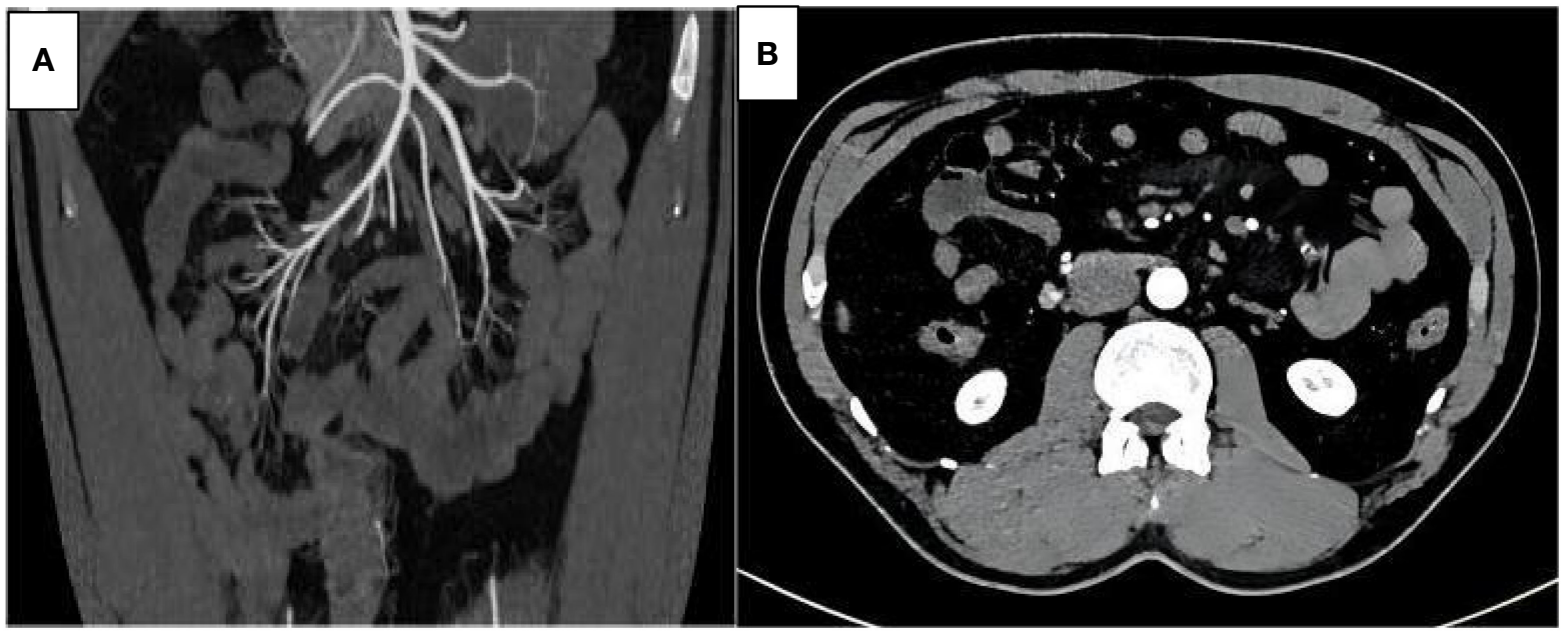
Three-dimensional enhanced abdominal CT scan (A transverse view, B coronal view) 20 months after surgery: no recurrence of small intestinal lipomatosis was observed.
